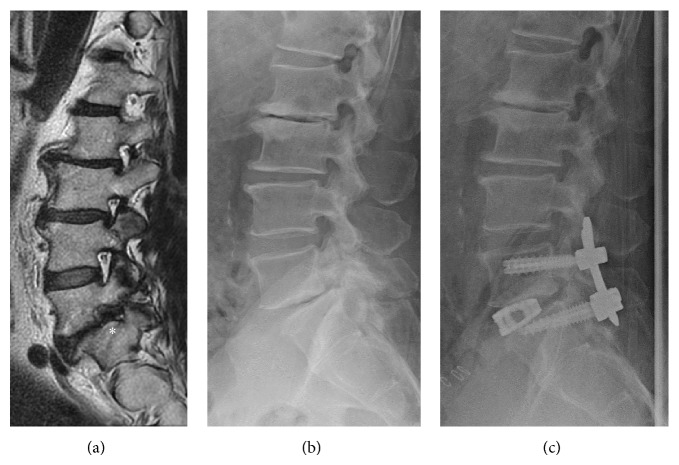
(a) Sagittal T2-weighted MRI showing foraminal stenosis at L5-S1 (^*^). (b) X-ray image showing L5-S1 disk narrowing and L5 spondylolytic spondylolisthesis before surgery. (c) X-ray image after surgery.
